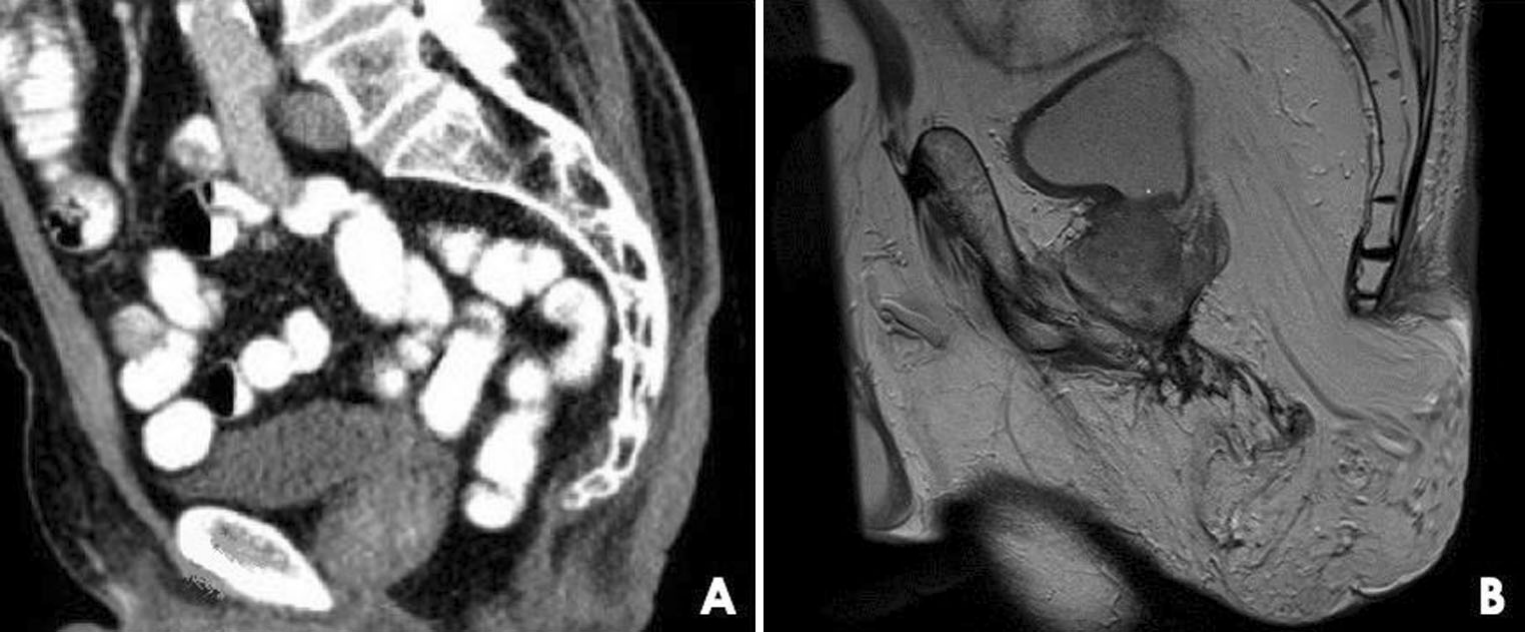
Two male patients after abdominoperineal resection with primary perineal closure for rectal cancer. a Computed tomography image, sagittal plane, showing descent of a small bowel loop with restricted mesenteric length. b Magnetic resonance image, sagittal plane, showing a large perineal hernia in which the hernia sac is filled with fully mobilized bulky omentum
